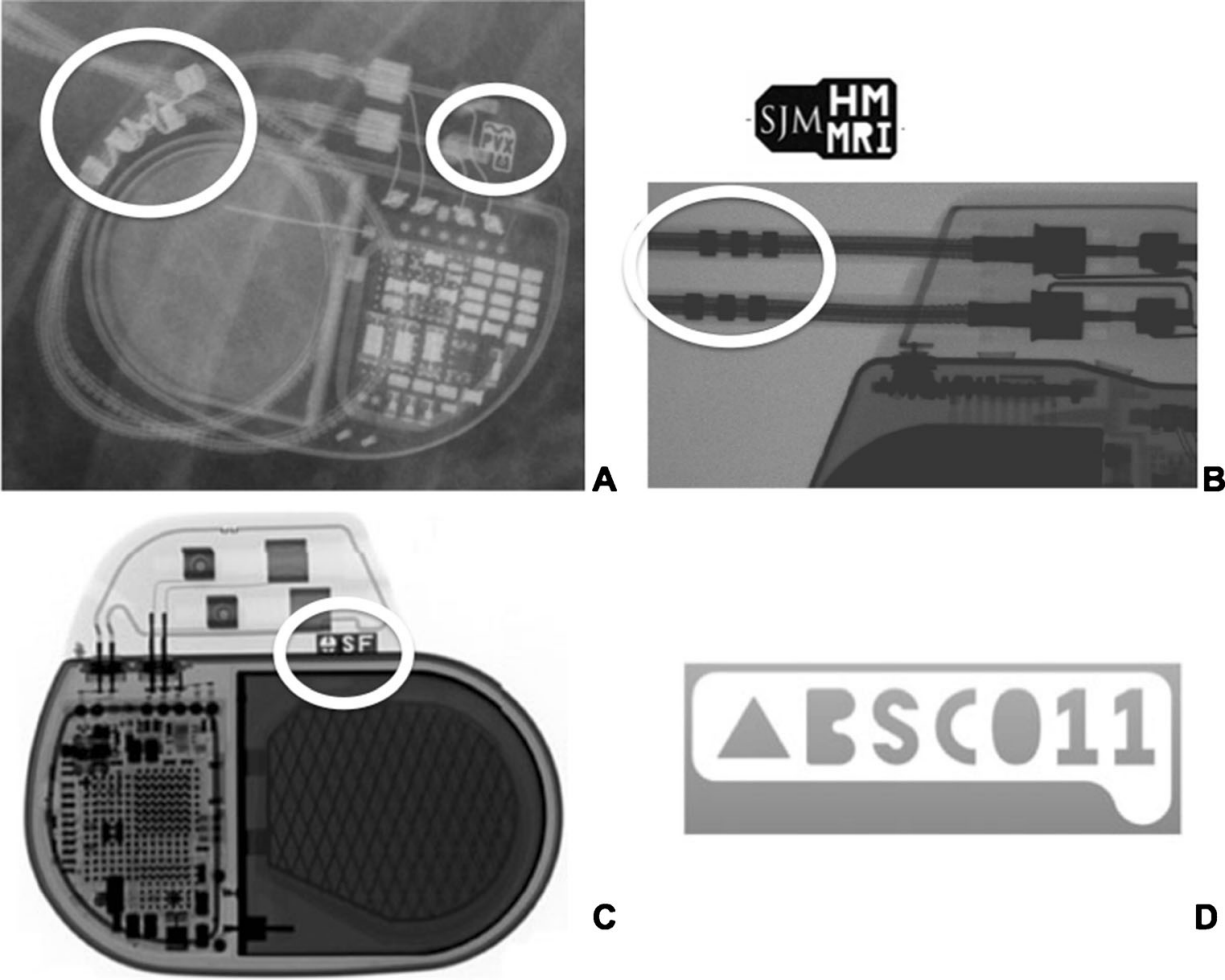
Vendor-specific markers on MRI conditional pacing systems as can be identified on routine chest X-rays. a Medtronic, b St. Jude Medical, c Biotronik, d Boston Scientific
